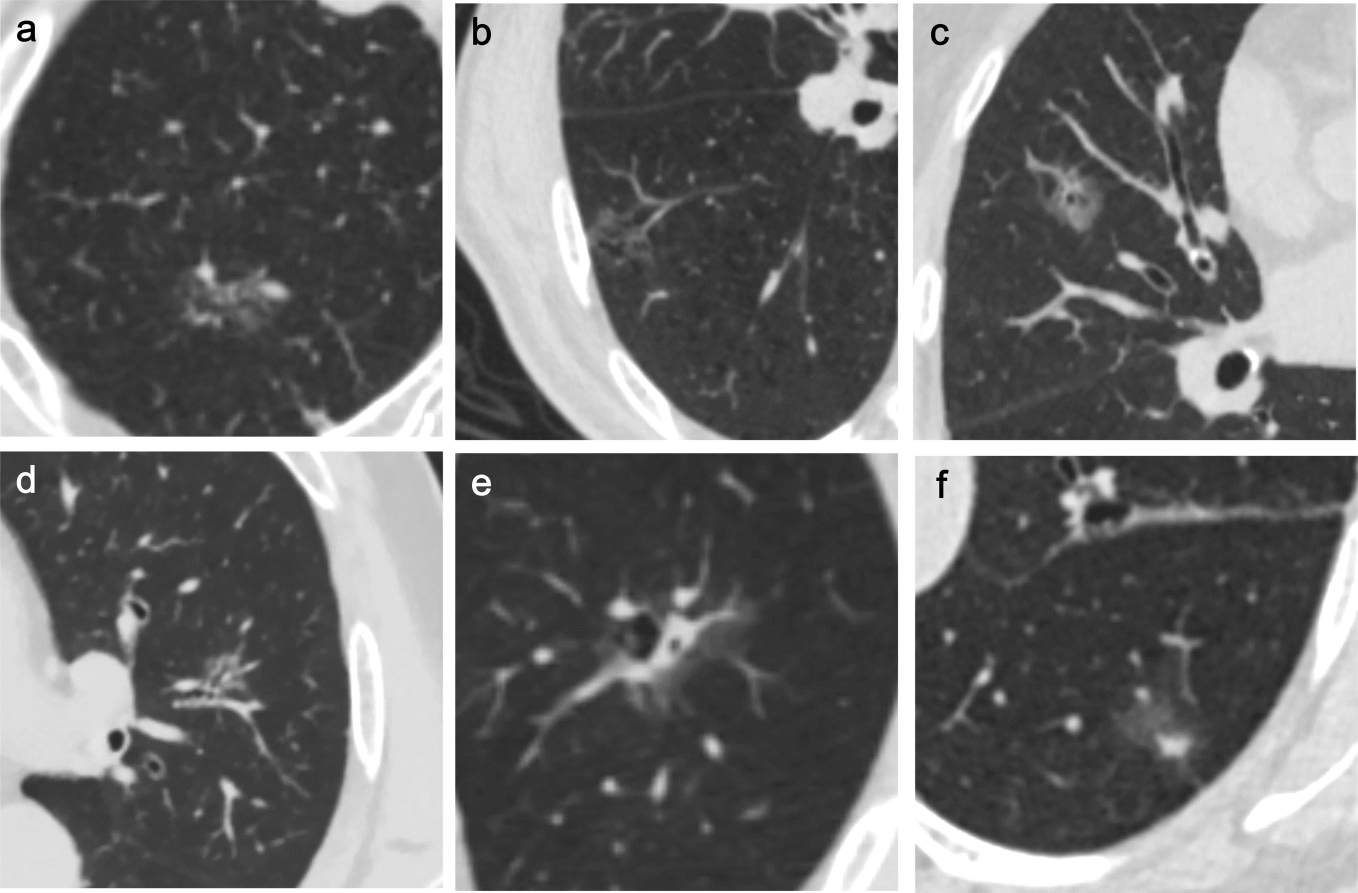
Independent risk factors for SSN growth in CT non-quantitative factor analysis. The six imaging features mentioned in the article are as follows: (A) lobulated sign, (B) vacuole sign, (C) vascular sign, (D) air bronchogram, (E) vacuole sign in PSN, (F) size of the solid component in the nodule.
